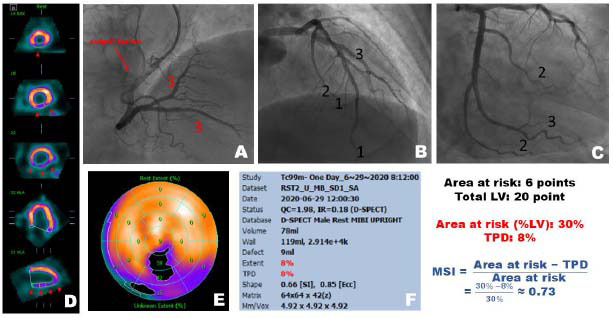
One example combining Coronary Angiography (A-C) and SPECT (D-F) for Myocardial Salvage Index Calculation. The red triangle on the left side of the MPI indicates that the patient’s MI is located in the posterior-inferior wall (D), and the coronary angiography on the right side suggests that the culprit lesion (indicated by a red arrow in A) is located in the proximal RCA
